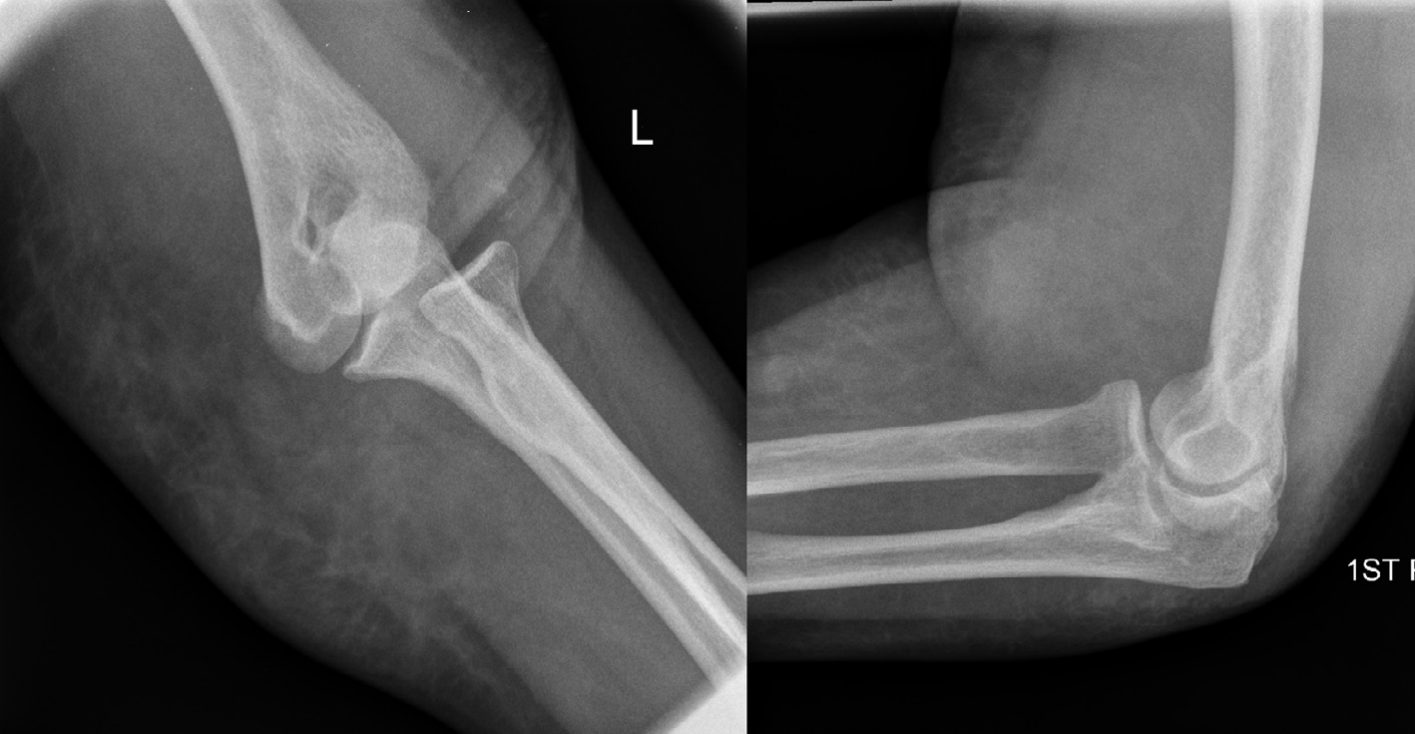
Elbow AP and lateral radiographs following closed reduction.
